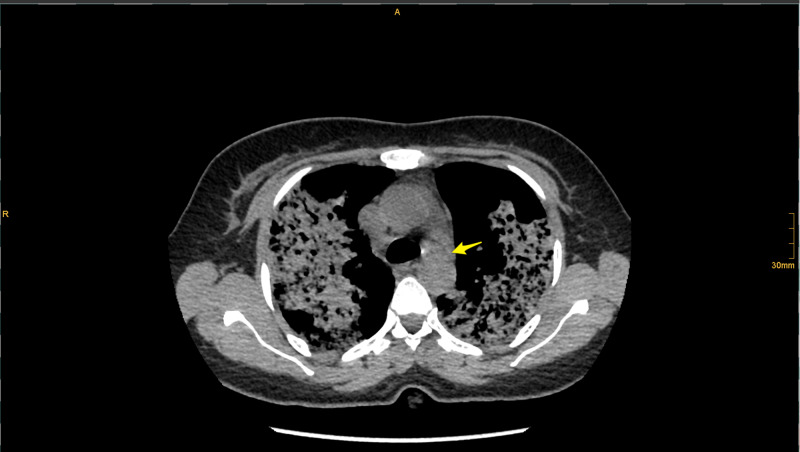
CT chest (Mediastinal window) The evaluation is limited but there are a few paratracheal lymph nodes (yellow arrow).
